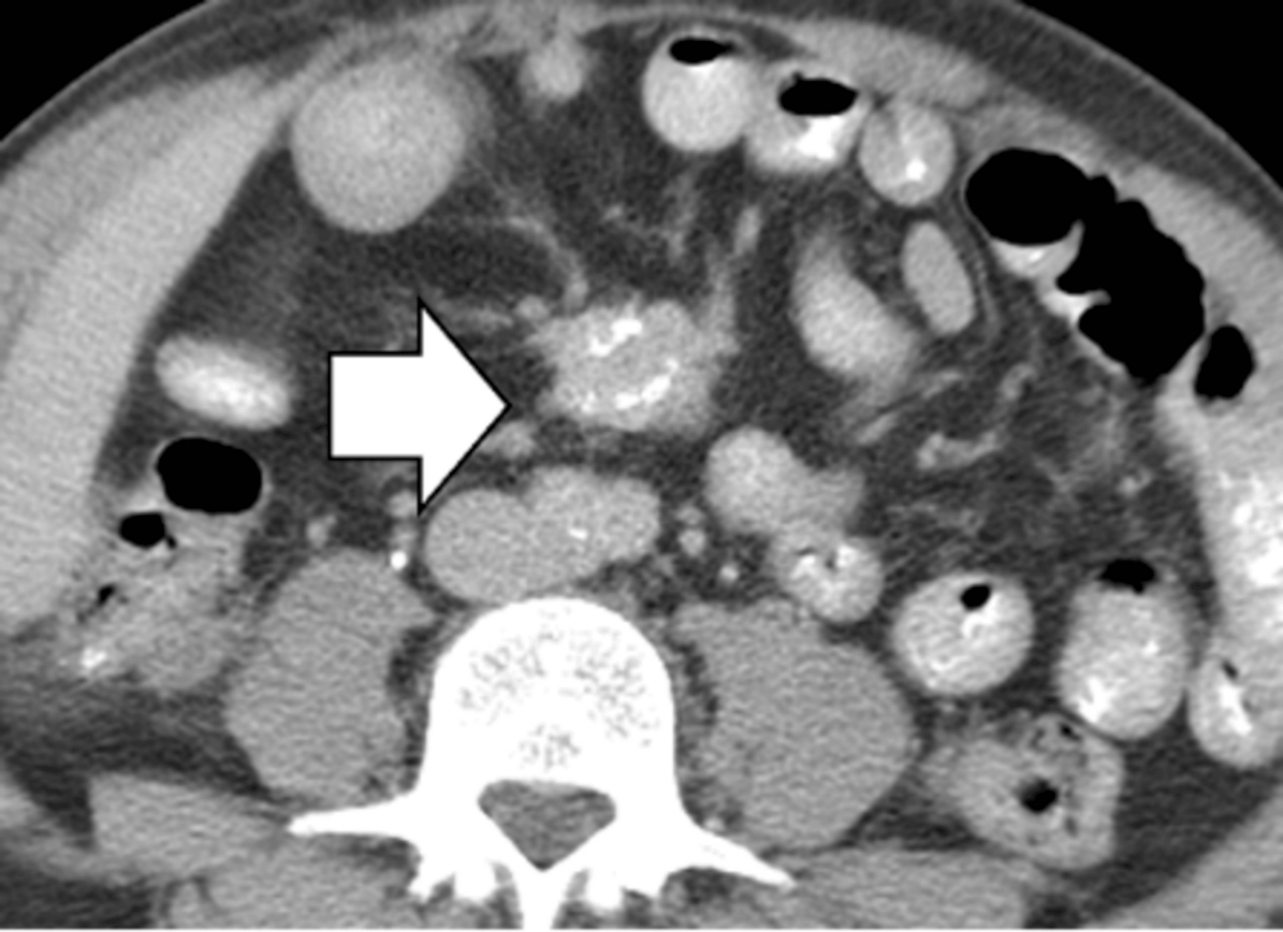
Sclerosing mesenteritis. CT image shows an ill-defined, infiltrating soft-tissue mass (white arrow) in the root of the mesentery with associated calcification and desmoplastic reaction.
